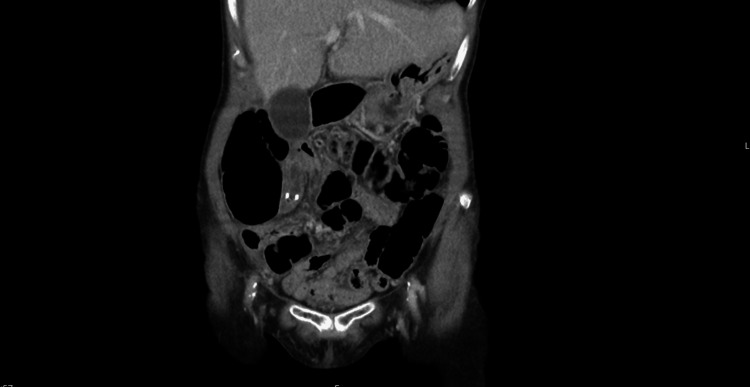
Coronal CT of the abdomen and pelvis with intravenous contrast demonstrating the same fluid-filled appendiceal lesion with mural calcification, in continuity with the cecum.
